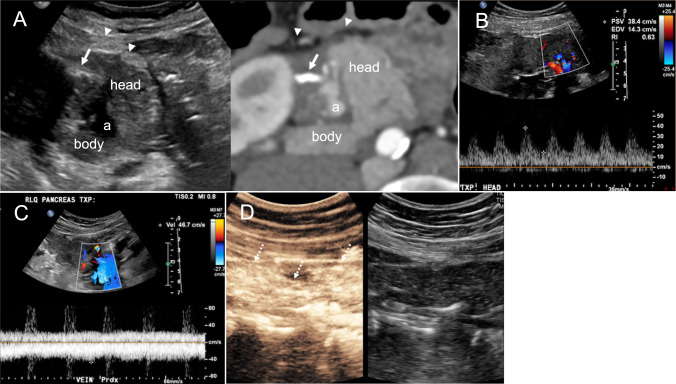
Ultrasound findings of a normal pancreas graft. Grayscale ultrasound with the corresponding computed tomography image for comparison A demonstrates a homogeneous hypoechoic pancreas graft head and tail surrounded by echogenic fatty tissue (arrowhead). The superior mesenteric vein (a) is seen as an anechoic structure near the pancreatic head. The echogenic line (arrow) corresponds to a surgical staple. Spectral Doppler of the artery B shows normal arterial waveforms with rapid systolic upstroke and continuous diastolic flow, and normal monophasic venous waveforms C in portal venous drainage. On contrast-enhanced ultrasound (D), the pancreas graft shows homogeneous enhancement within 10–20 s after contrast injection (dashed arrow)
